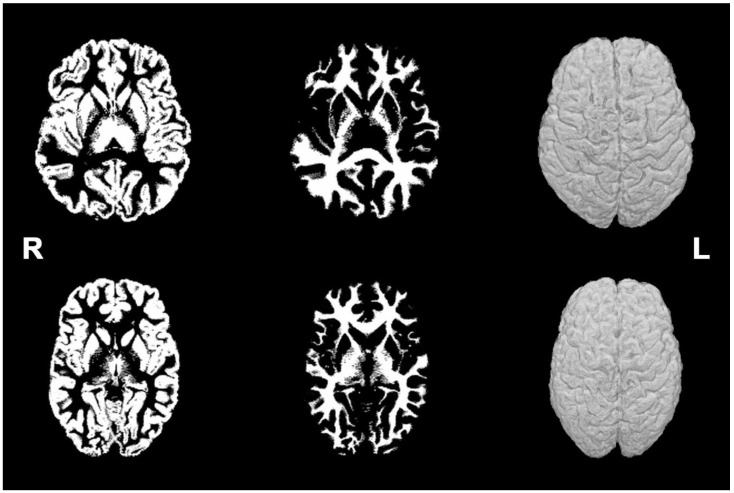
Representative MRI brain images for two phenotypes (gray matter, white matter, and rendered 3D surface). (Top row): normal subject. (Bottom row): Cluster 1 or “small brain phenotype” subject.
